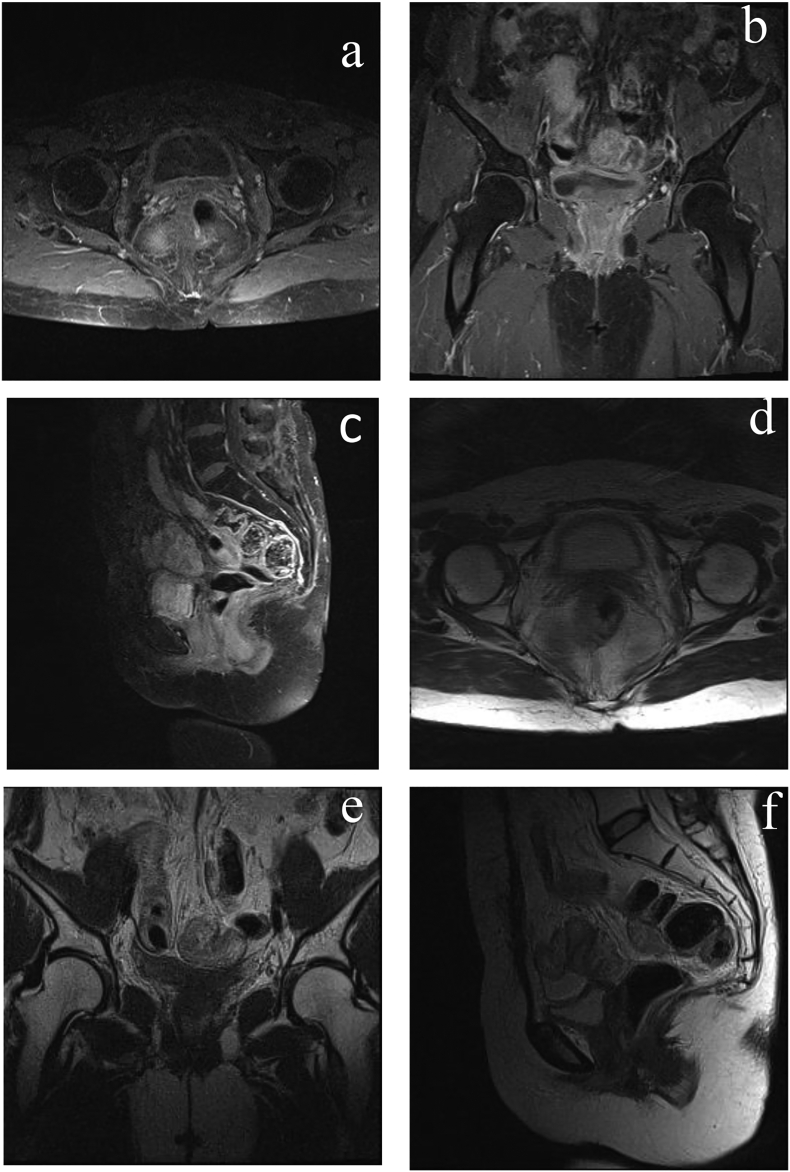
(a to f). Abdominal magnetic resonance imaging with contrast taken after radical hysterectomy and series radiotherapy was completed. Seven months followed-up.
